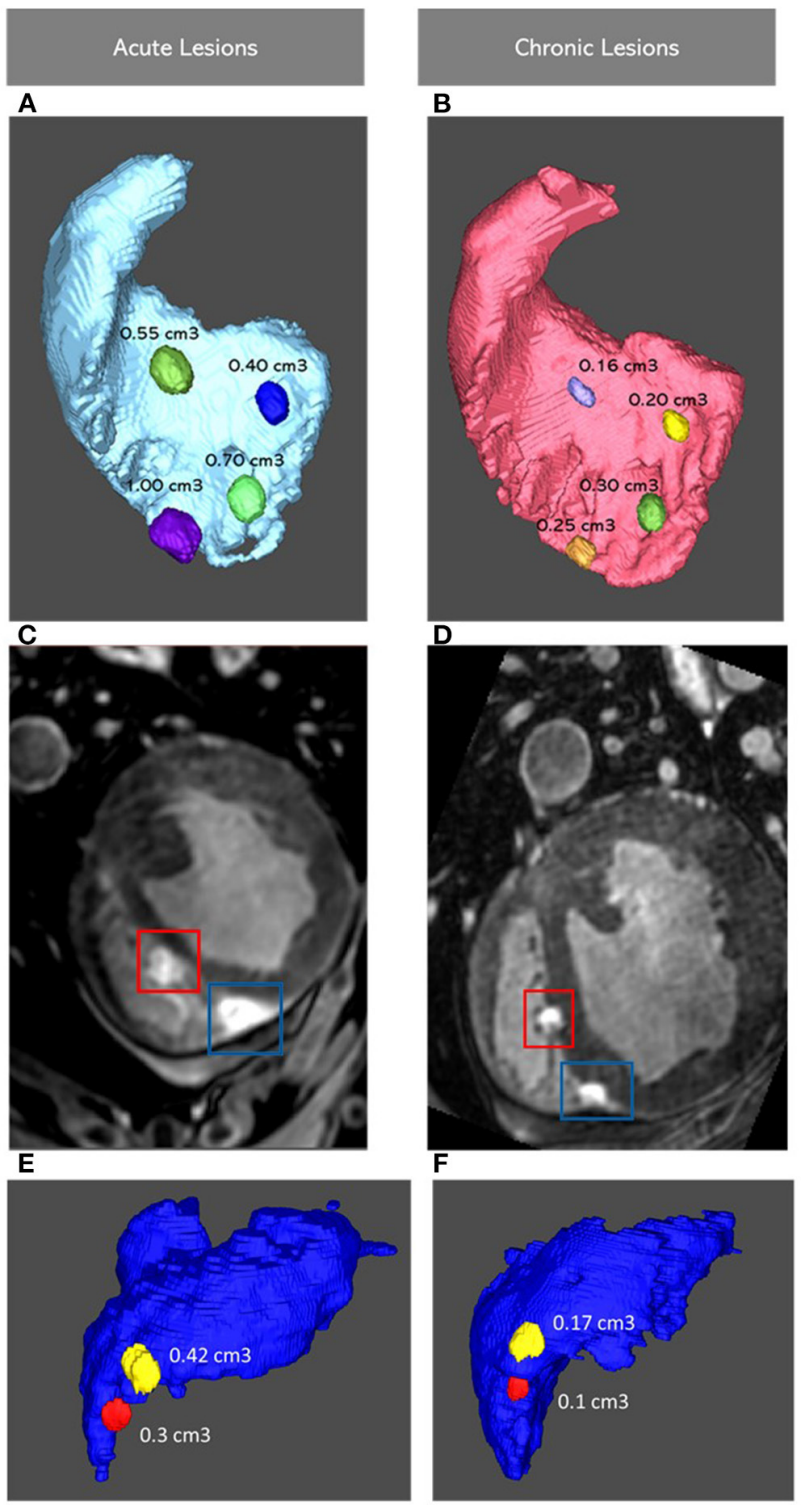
Images Showing four Lesions Represented on Corview in the RV Septum in the Acute (A) and Chronic (B) Settings, with corresponding LGE-MRI Images of the Lower Two Lesions (C,D). Images Showing two Lesions in The RV Free Wall (E,F). RV: Right Ventricle; LGE-MRI: Late Gadolinium Enhancement Magnetic Resonance Imaging.
